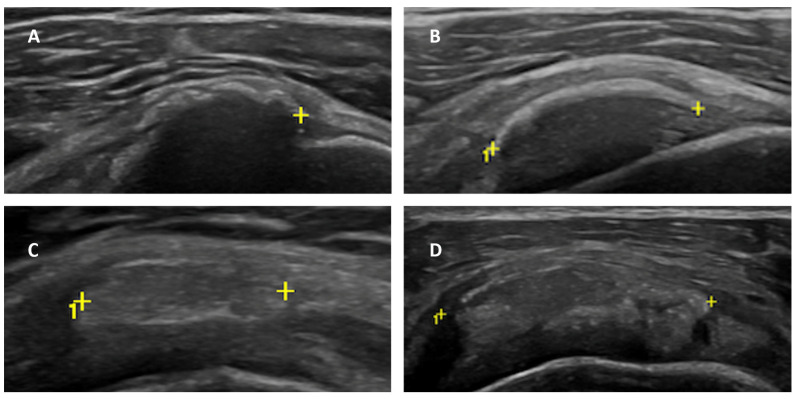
Calcific maturation stage: stage 1 (A)—arc-shaped with strong posterior shadowing); Stage 2 (B)—fragmented or punctate with partial posterior shadowing); Stage 3 (C)—nodular without posterior shadowing); Stage 4 (D)—nodular with cystic degenerative areas and without posterior shadowing). Symbol (+) represents measurement landmarks in ultrasound images.
